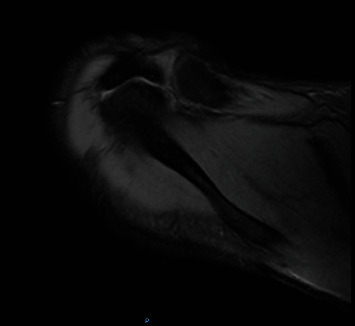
T2-weighted axial magnetic resonance image of meso-type Os acromiale.
